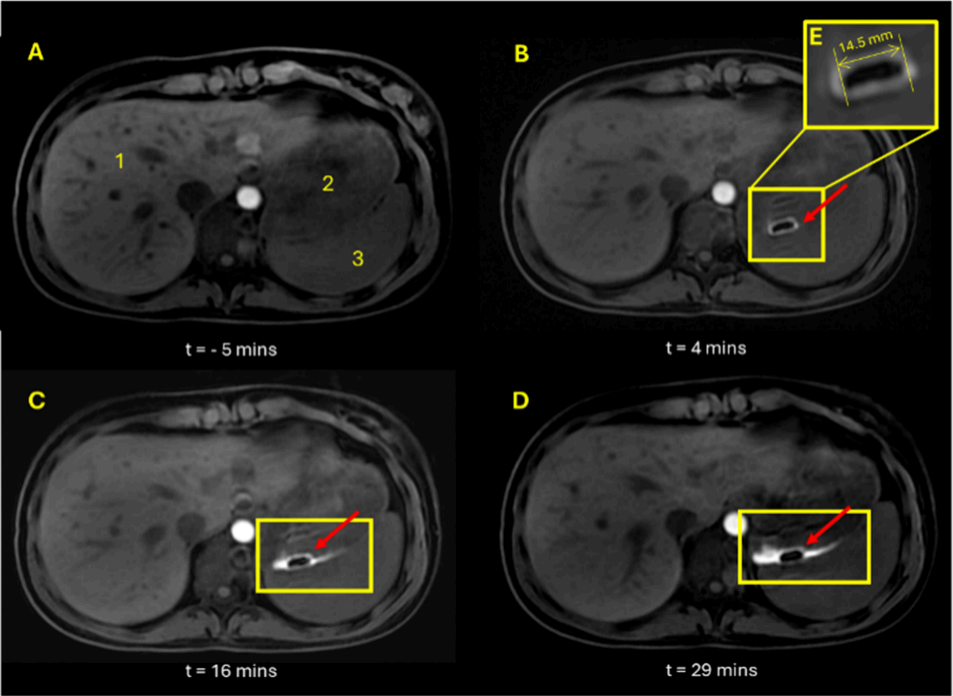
Axial, 3D LAVA MRI images of abdomen of participant
